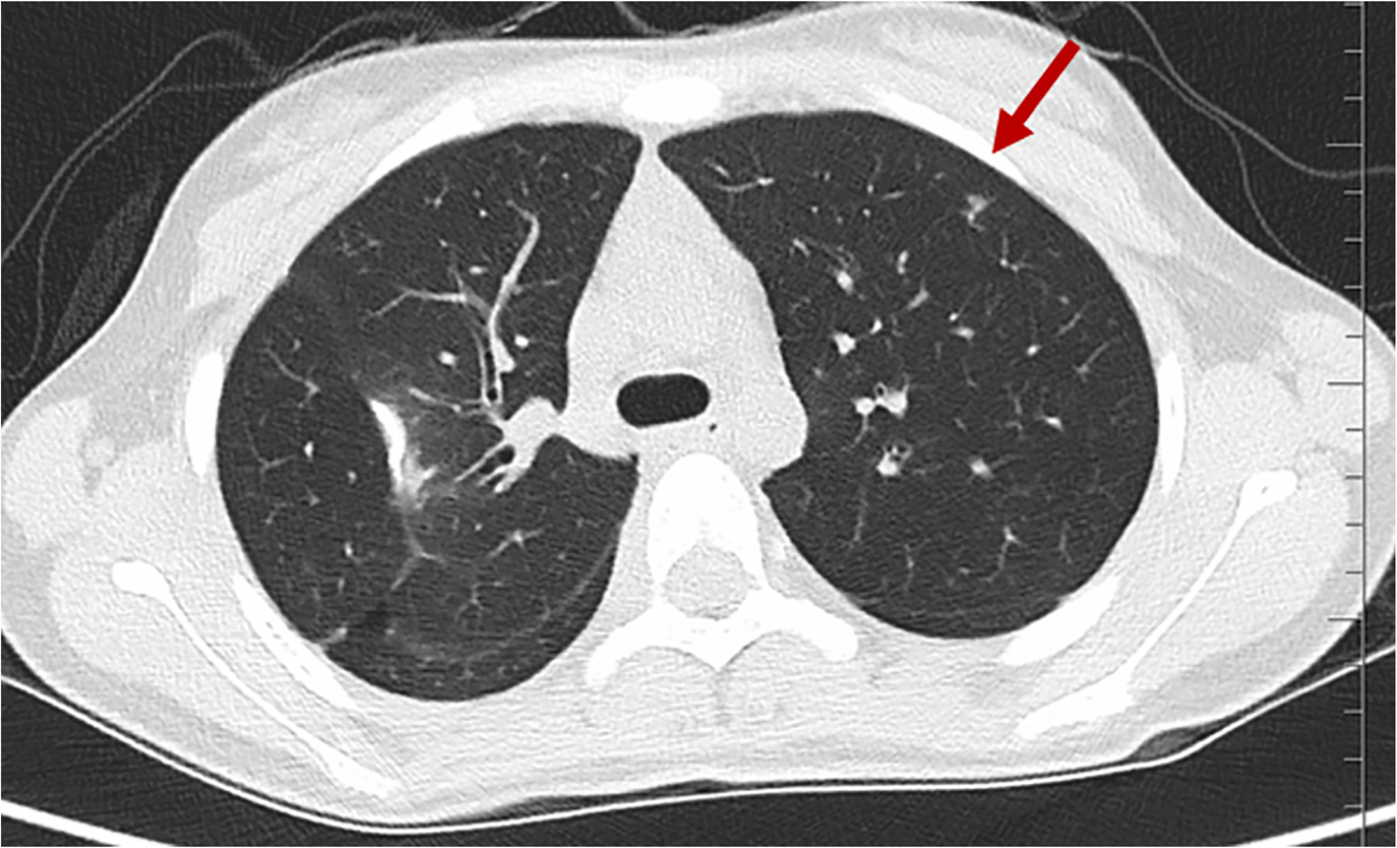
A missing nodule shown on chest CT (red arrow) two weeks after metastasectomy. (A surgical scar can be observed on the right lobe of the lung. CT = computed tomography)
